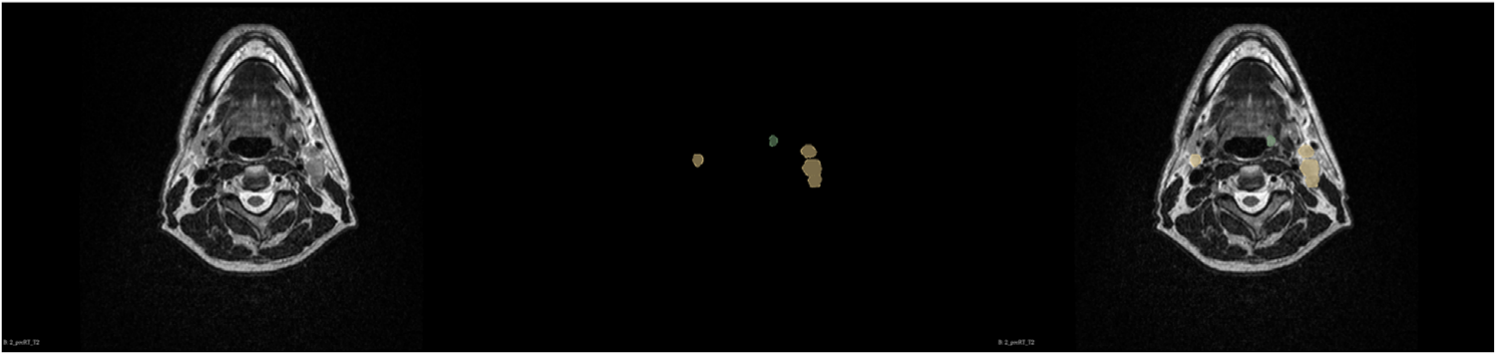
Example of the head and neck MRI data from a patient in the HNTS‐MRG. Green represents tumor GTVp, and yellow represents GTVn. GTVn metastatic lymph nodes; GTVp, primary gross tumor volume; HNTS‐MRG, head and neck tumor segmentation for MR‐guided applications.
